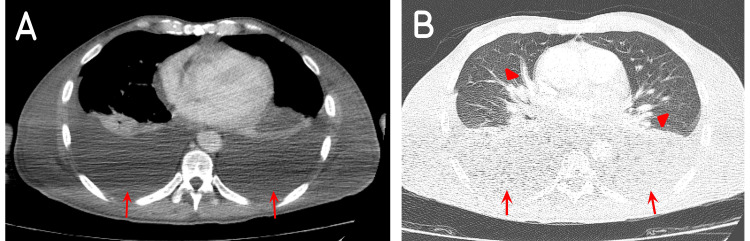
CECT of the chest in Case 1 showing (A, B) a moderate amount of bilateral pleural effusions (red arrows) and (B) congested lung parenchyma (red arrowheads). A, mediastinal view; B, lung view CECT: contrast-enhanced computed tomography
